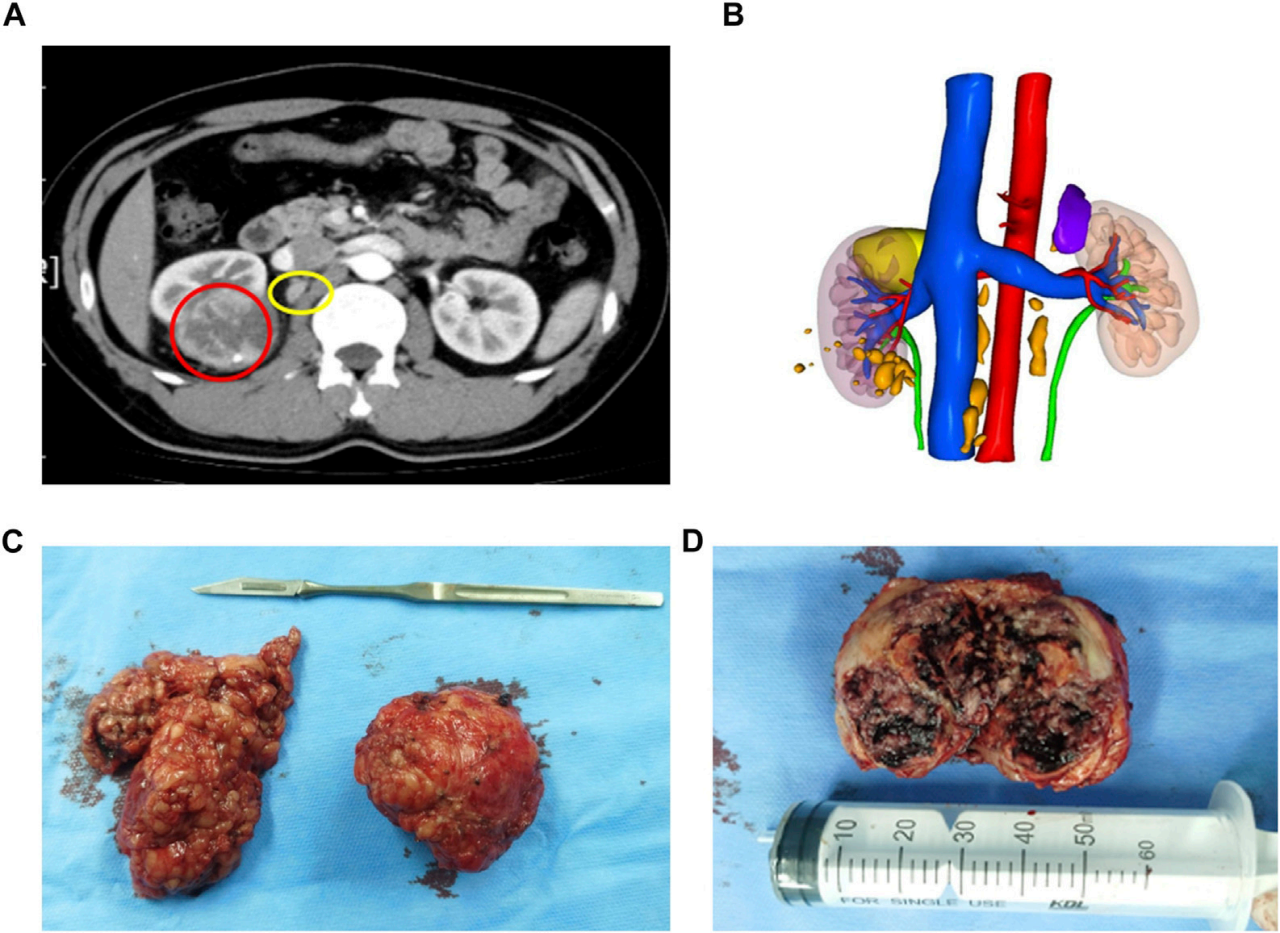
Conventional CT and 3D reconstruction demonstrated a tumor and enlarged lymph nodes. (A) Abdominal CT scan detected a solid mass (6 cm in diameter) at the right kidney (red circle) and multiple enlarged lymph nodes (yellow circle). (B) 3D reconstruction of the urinary system which includes lesions (tumor: yellow; enlarged lymph nodes: orange), kidneys, collective system, and blood vessels. (C) A well-circumscribed solid mass on the right and multiple enlarged hilar lymph nodes scavenged on the left. (D) Cut surface of a well-encapsulated tumor lesion showing gray-white or gray-yellow and a large area of necrosis, and the tumor was 6 × 5.5 × 5 cm^3^ in size.
